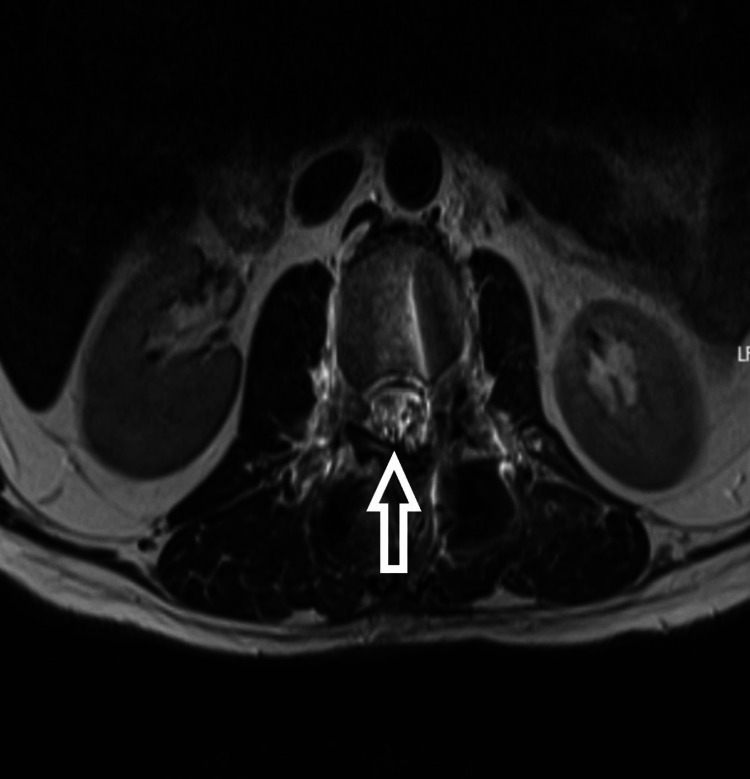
Immediate post-operative axial MRI
